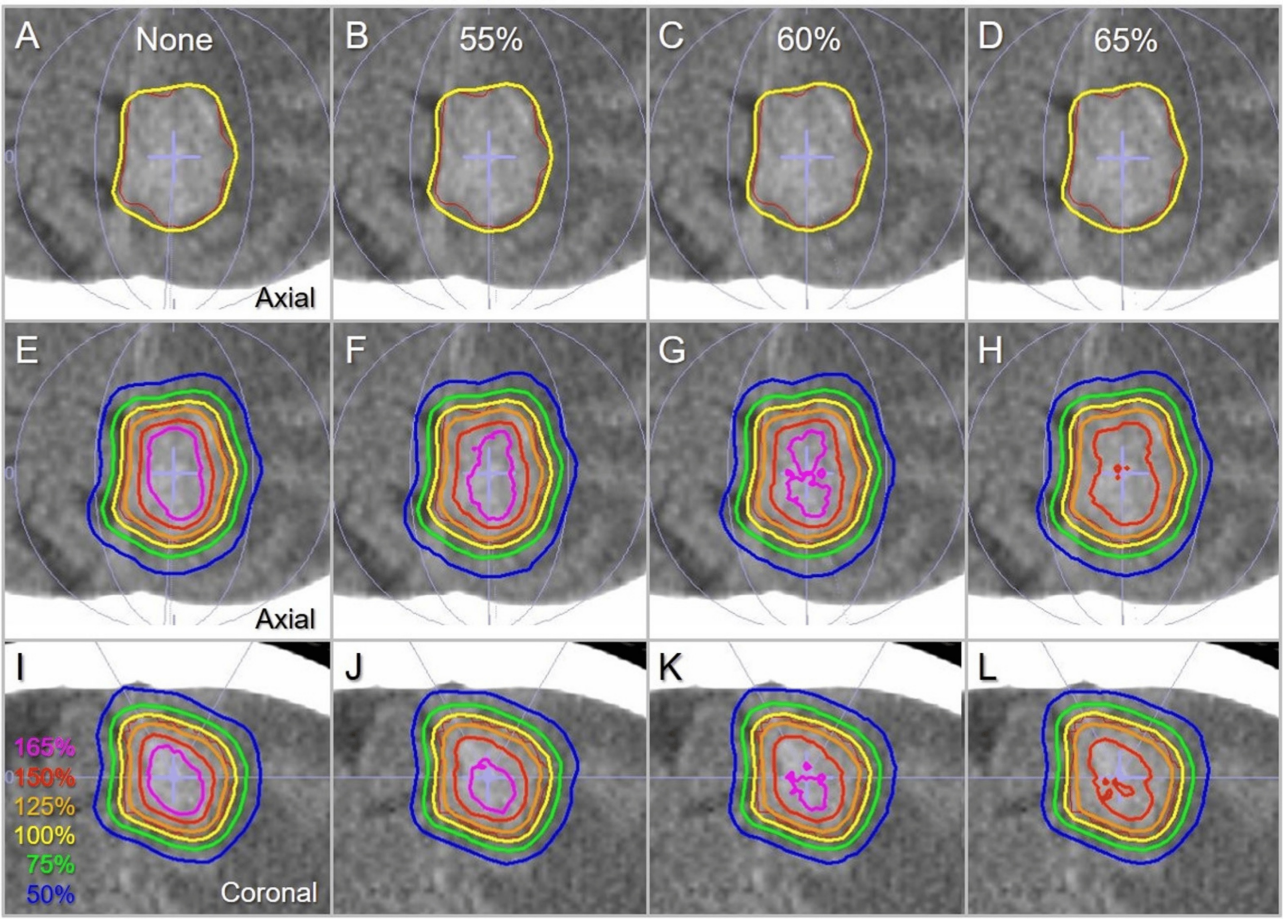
Comparison of the dose distributions for a GTV of 7.14 cc. The images show computed tomographic scans of a patient with a brain metastasis (BM) located in the left frontoparietal lobes (A-F), with superimposed representations of the GTV outline (red), arc arrangements (light purple), and representative isodose lines from the None, 55%, 60%, and 65% plans. Axial views (A-H) and coronal views (I-L) are displayed. The isodose lines are presented as relative values, using the GTV D_V-0.01_ cc (D99.86%) as 100% (yellow). After rescaling the GTV coverage, the isodose values of the GTV D_V-0.01 cc_ relative to the D_0.01 cc_ (100%) are 55.4%, 57.7%, 58.4%, and 62.0% in the None, 55%, 60%, and 65% plans, respectively. GTV: Gross tumor volume; BM: Brain metastasis; D_V-0.01 cc_: Minimum dose to a target volume (TV) minus 0.01 cc; D_0.01 cc_: Minimum dose to 0.01 cc of a TV, receiving a near-maximum dose.
